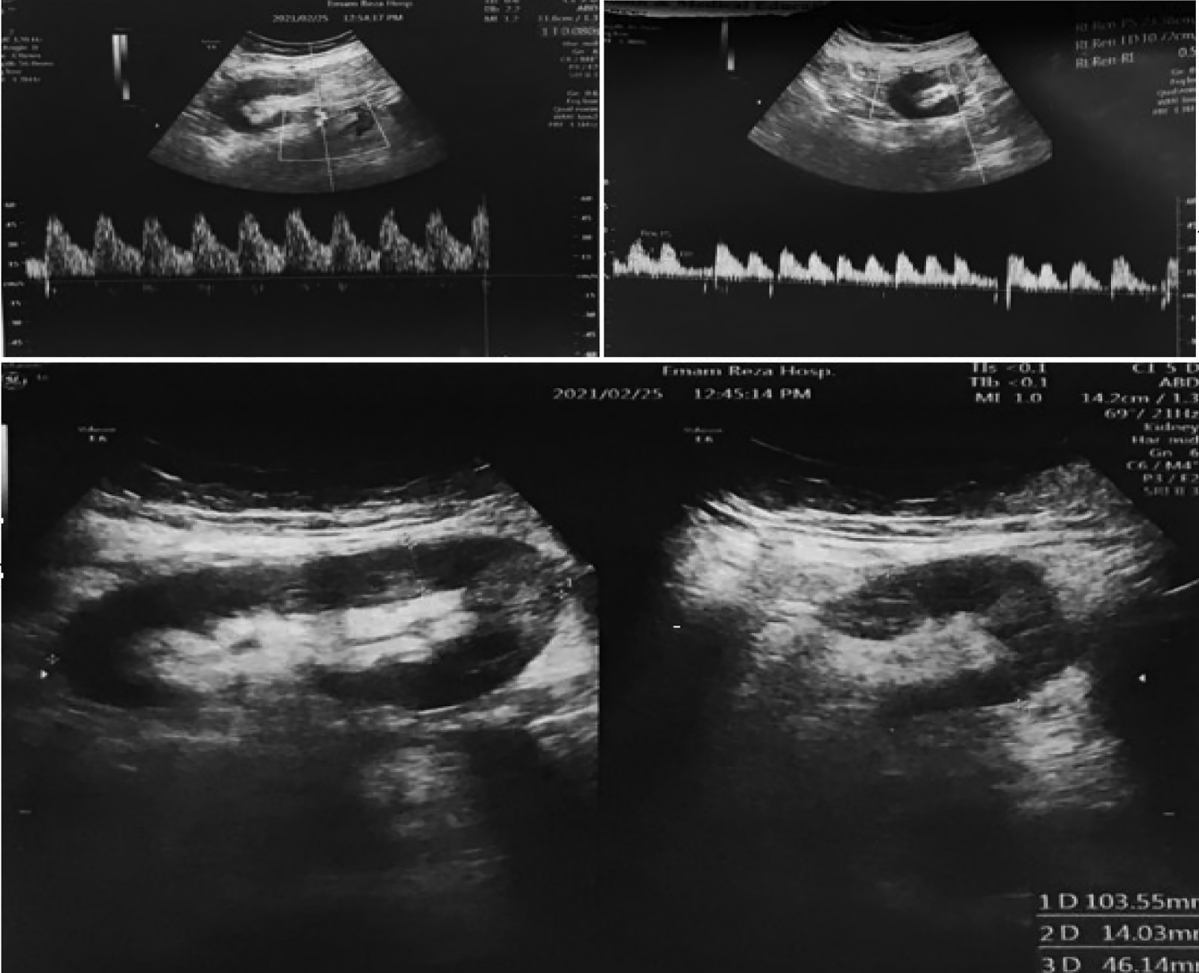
Duplex ultrasound of the patient; showing decreased vascular perfusion, there is no signs of artery or vein stenosis of the renal transplant.
